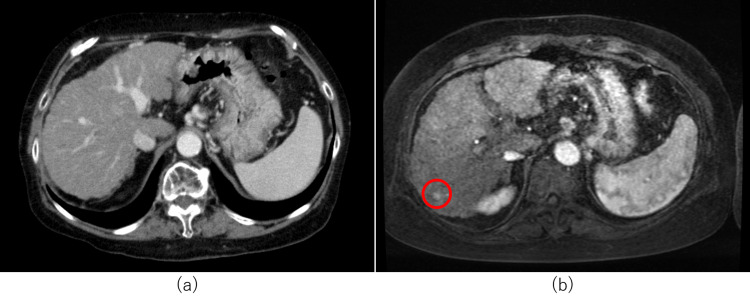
CT and MRI findings of Case 2 (a) Contrast-enhanced CT image at admission: a liver cirrhosis pattern is seen. (b) MRI image with gadolinium ethoxybenzyl diethylenetriaminepentaacetic acid after nine months: there is no progression of hepatic atrophy, but a high/low-pattern hepatocellular carcinoma is seen in liver segment 6 (red circle). Liver hardness is equivalent to F4 CT: computed tomography; MRI: magnetic resonance imaging
